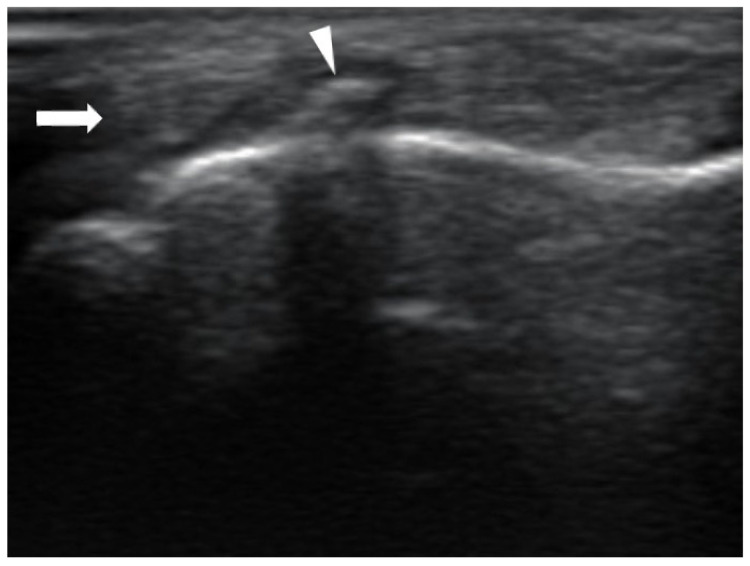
Ultrasound image of the little finger in longitudinal view. The arrowhead points to the bony spike arising from the fracture site of the proximal phalanx. The arrow indicates the flexor tendon being incarcerated and mechanically impinged by the bony protrusion.
